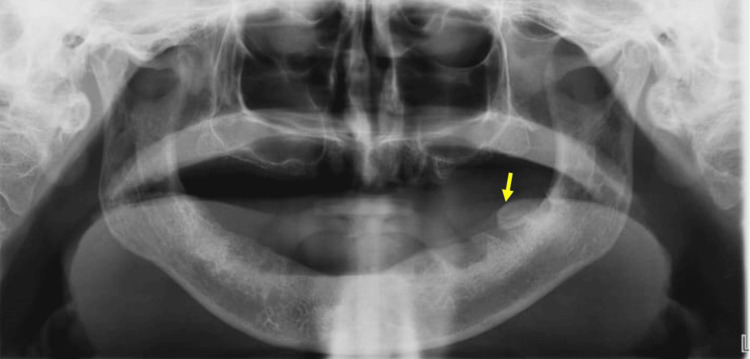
Orthopantomography revealed a radiopaque protuberance in the edentulous mandibular second molar region (yellow arrow).
